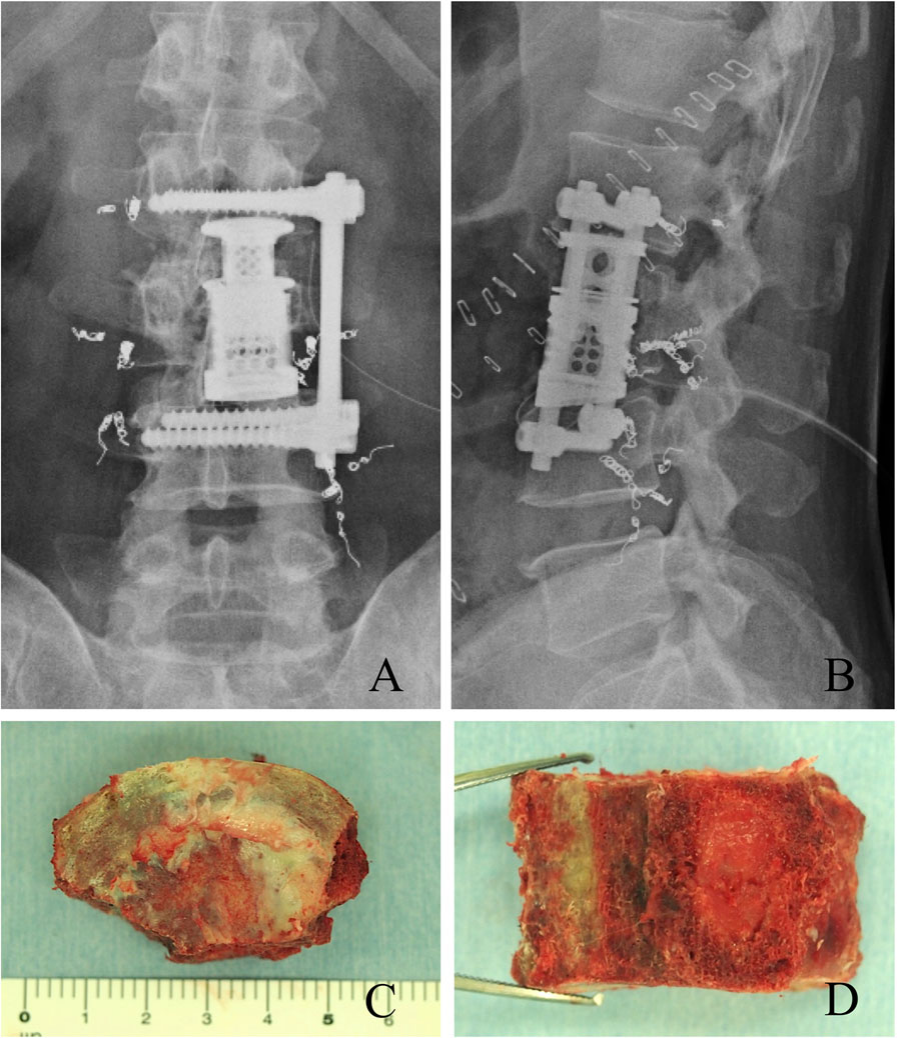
Frontal (a) and lateral (b) view of postoperative radiographs. Top view (c) and a cross-sectional view (d) of the resected L3 vertebral body
